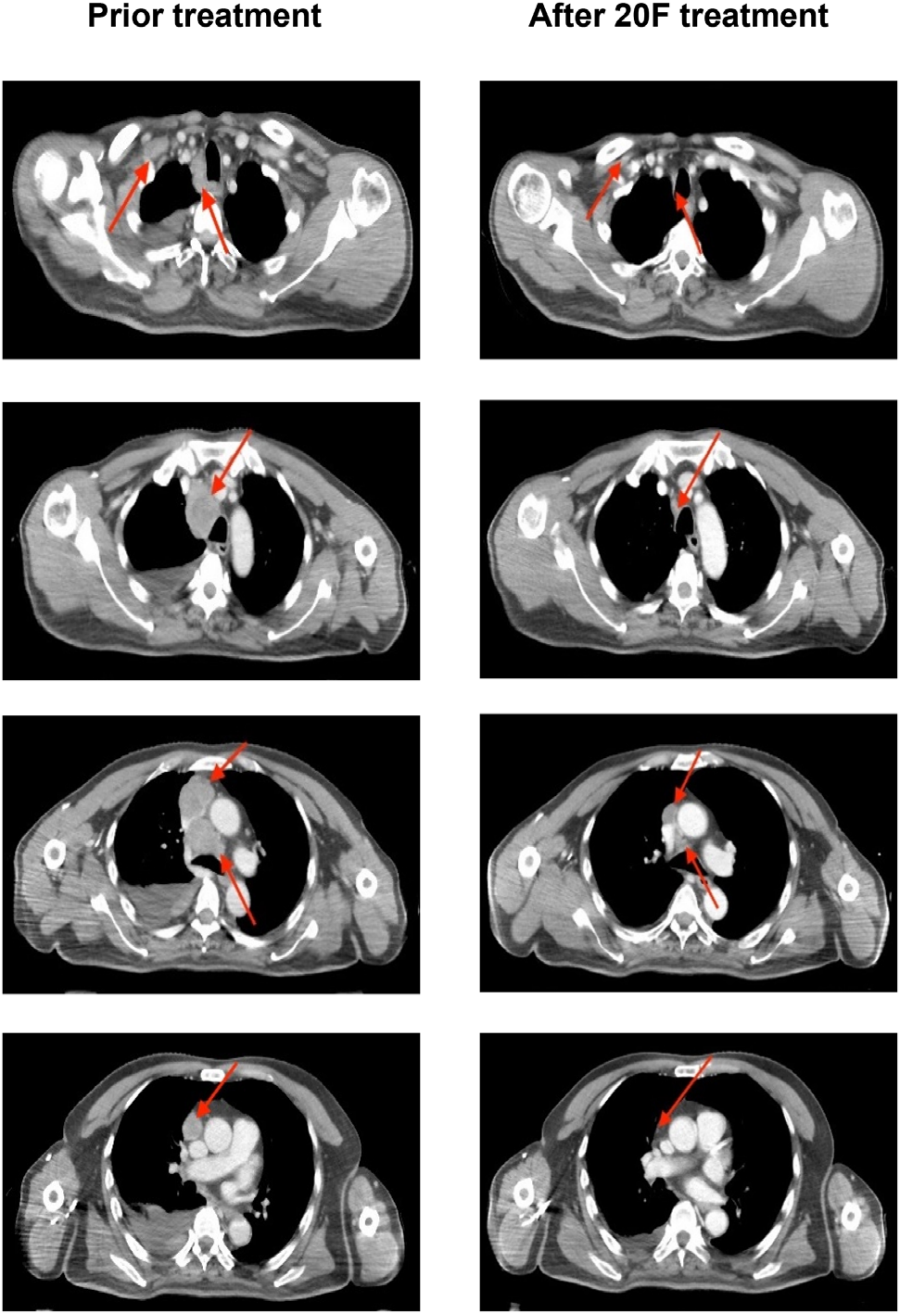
Computed tomography (CT) images of the patient before radiotherapy and after 20 fractions of radiotherapy. The first column displays the CT images taken prior to radiotherapy, while the follow‐up CT images after 20 fractions (F) of radiotherapy are shown in the second column. All images are presented using a window width of 400 and a window level of 800. The tumor area is indicated by red arrows in both the pretreatment and post 20F treatment images.
